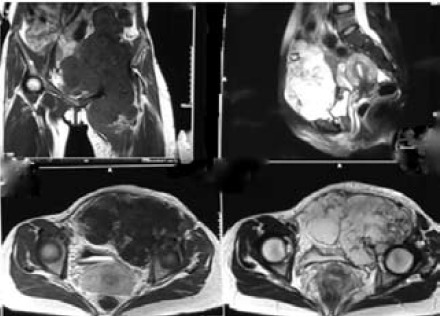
Fig. 4: Plain radiographs of pelvis (right) and left hip (left) - after -reconstruction F4:
